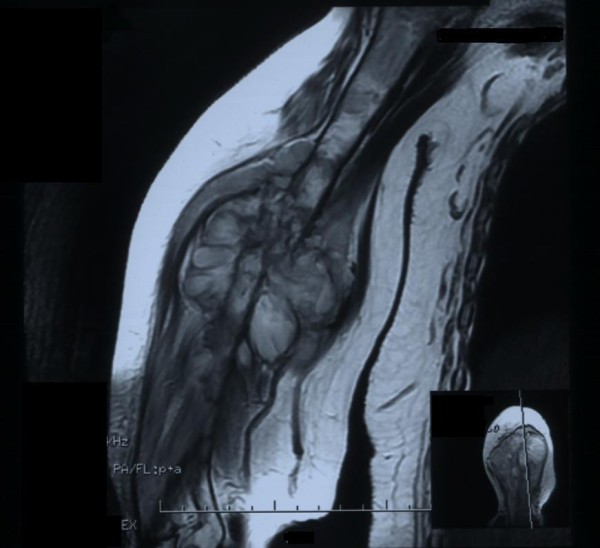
Magnetic resonance imaging scan showing extensive soft tissue mass with bone destruction of the left humerus.
